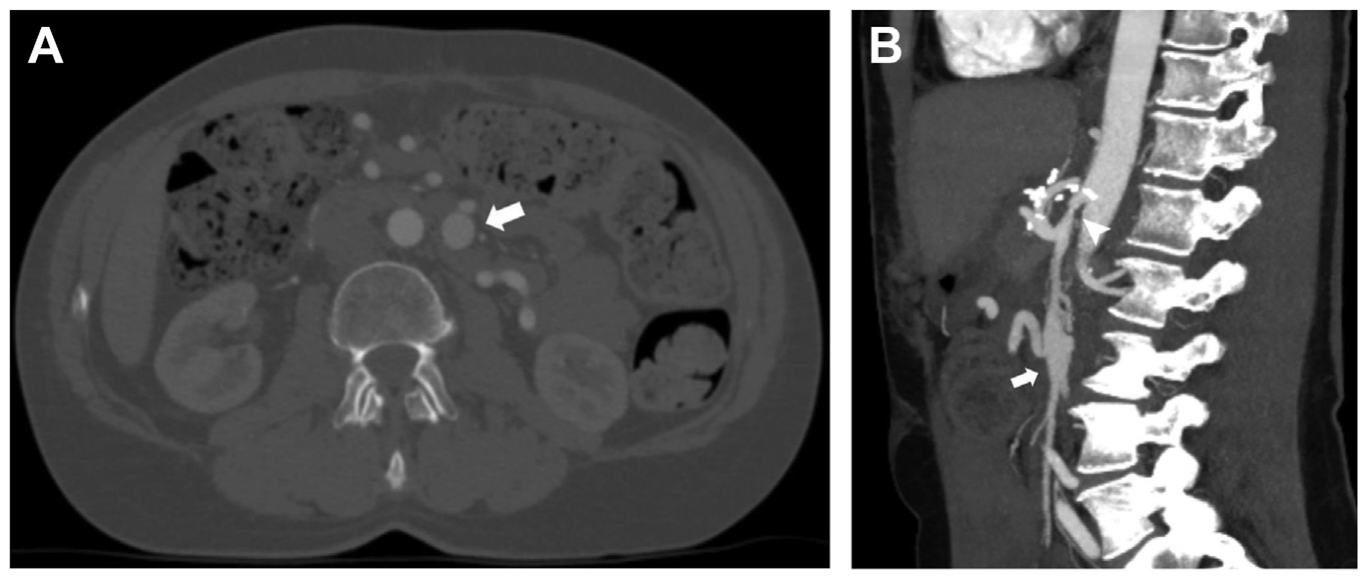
Postoperative computed tomography angiogram obtained at 6-month follow-up. A, Axial computed tomography angiogram cut depicting superior mesenteric artery (SMA) aneurysm, 1.8 cm in maximum diameter (*white arrow*). B, Sagittal computed tomography angiogram cut demonstrating resolved compression of celiacomesenteric trunk (CMT; *white arrowhead*) and stable SMA aneurysm (*white arrow*).
